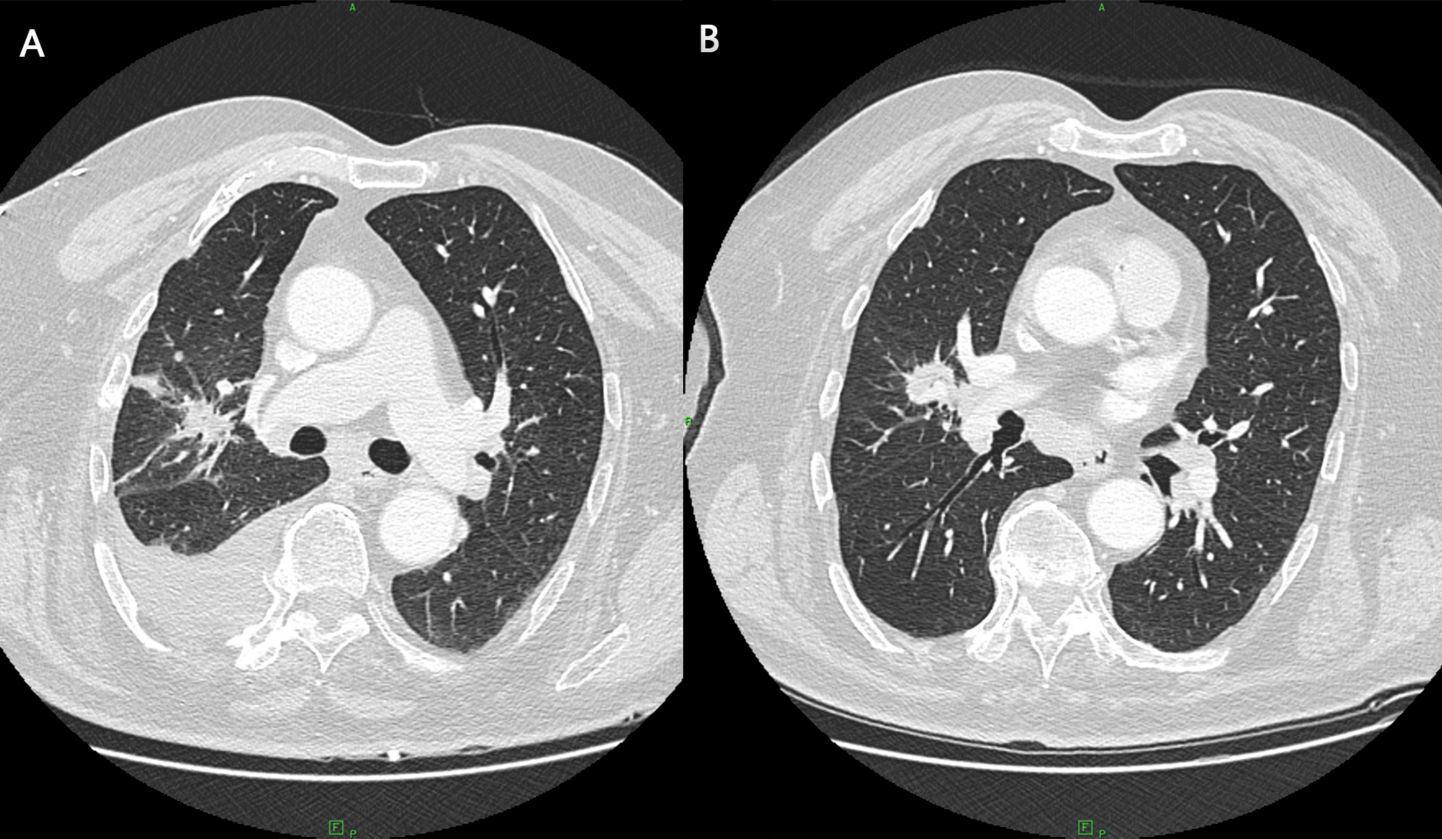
Postoperative (A) and preoperative (B) axial CT scans showing right parahilar lesion with irregular margins and plural fluid post VATS. (A) The parahilar lesion demonstrated irregular, fibrotic-appearing margins and (B) persisted without significant regression after video-assisted thoracoscopic surgery (VATS). (B) A mild right-sided pleural effusion was also noted postoperatively, gradually decreasing on subsequent follow-up imaging.
